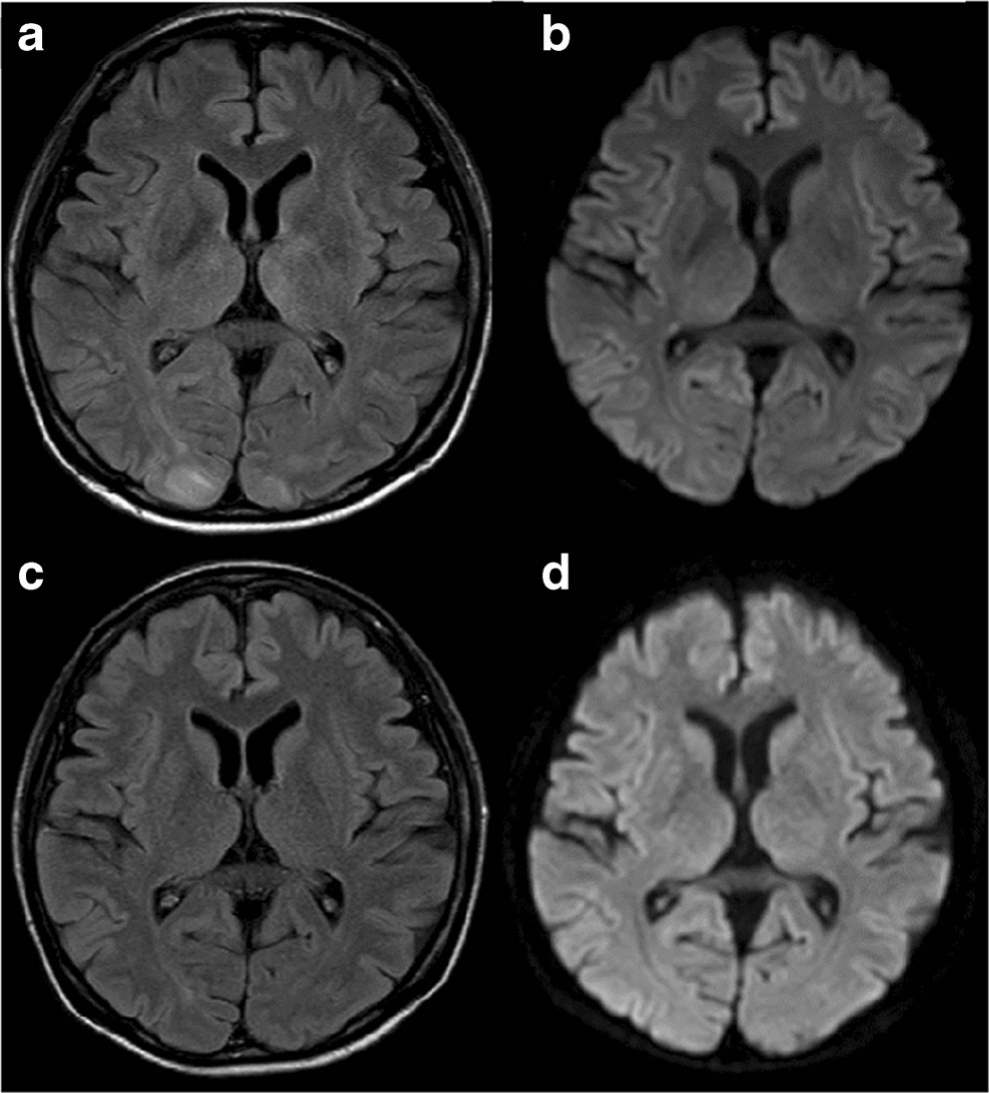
FLAIR (a) and DWI (b) on brain MR images at onset of PRES. FLAIR (c) and DWI (d) on brain MR images of the same patient after 3 weeks
